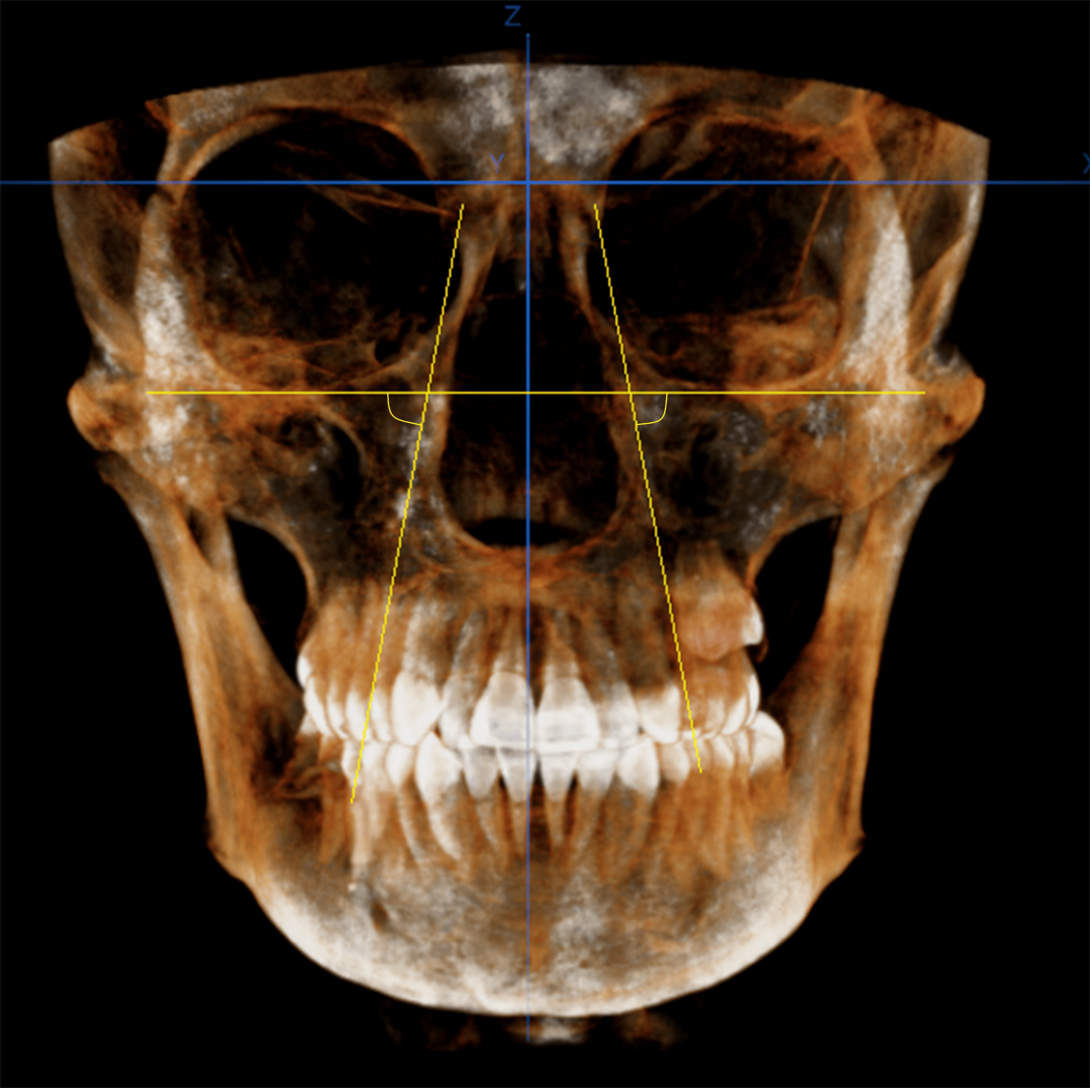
Measurement of the right and left maxillary first premolar buccolingual inclinations Yellow horizontal line: the line orbitale-orbitale; vertical yellow lines: maxillary first premolars axes; blue lines: cone-beam computed tomography orientation planes
